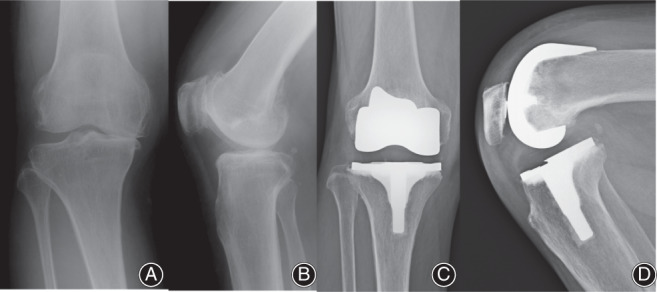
Radiographs of a right knee replaced with an anatomically shaped implant. (A) Preoperative anterior‐posterior view. (B) Preoperative lateral view. (C) Postoperative anterior‐posterior view. The joint line is maintained. (D) Postoperative lateral view.
